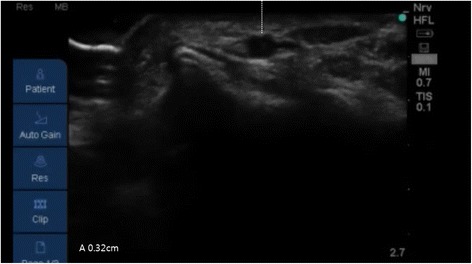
Measurement of the depth from skin of radial artery on the frozen image in ultrasound
